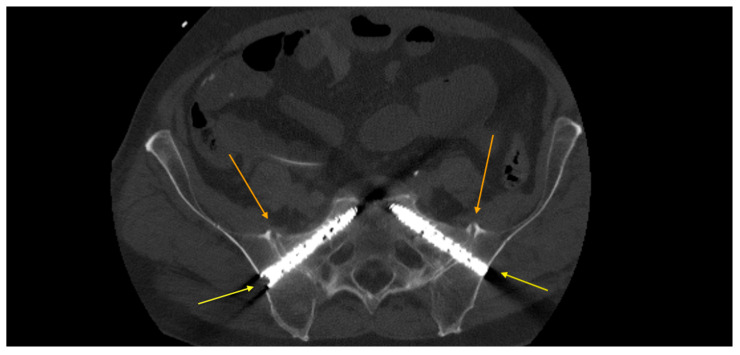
Sacroiliac-joint fusion via iFuse-TORQ implants^®^ shown in the CT-scan, axial view, with implants laying safely and entirely in the S1 corridor. Entry point is far posterior (yellow arrow) aiming ventrally toward the promontory as it is the area of the strongest bone formation. Notice the non-fused SI joints (orange arrows).
